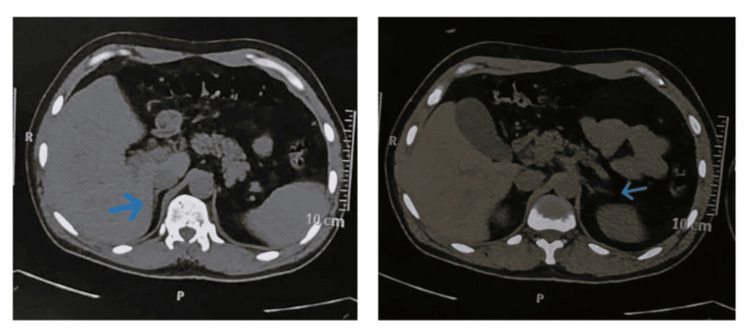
Computed tomography of the abdomen The computed tomography of the abdomen shows reduced volume of bilateral adrenal glands (arrows), suggestive of bilateral adrenal atrophy.
